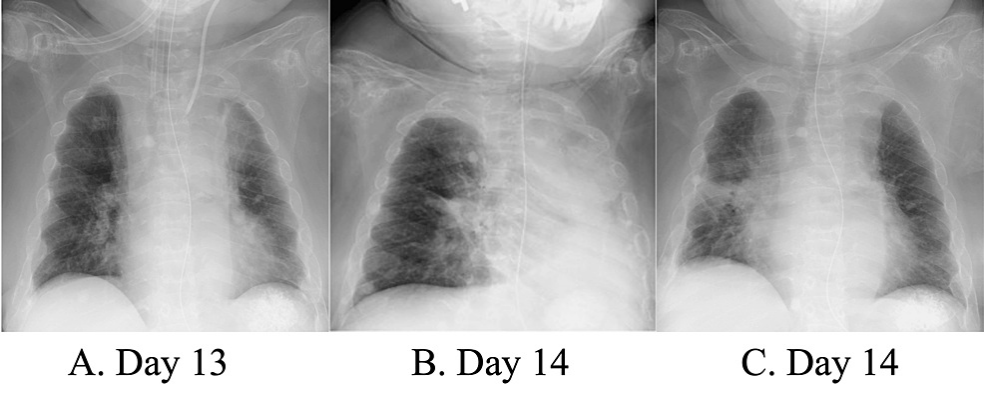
Serial changes in chest radiographs (A) A chest radiograph scan obtained on the 13th day after admission (just before extubation) showing no significant abnormal findings; (B) A chest radiograph scan obtained on the 14th day (a few hours after extubation) showing massive atelectasis in the left lung field; (C) A chest radiograph scan obtained a few hours after initiation of MI-E showing marked improvement of atelectasis in the left lung field, which was probably caused by sputum retention. MI-E: mechanical insufflation-exsufflation
